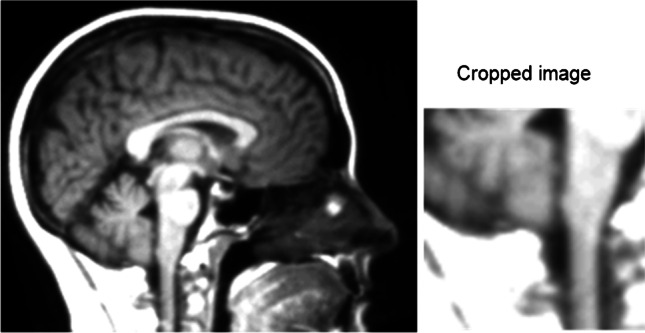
Example of a normal MRI misclassified as Chiari
